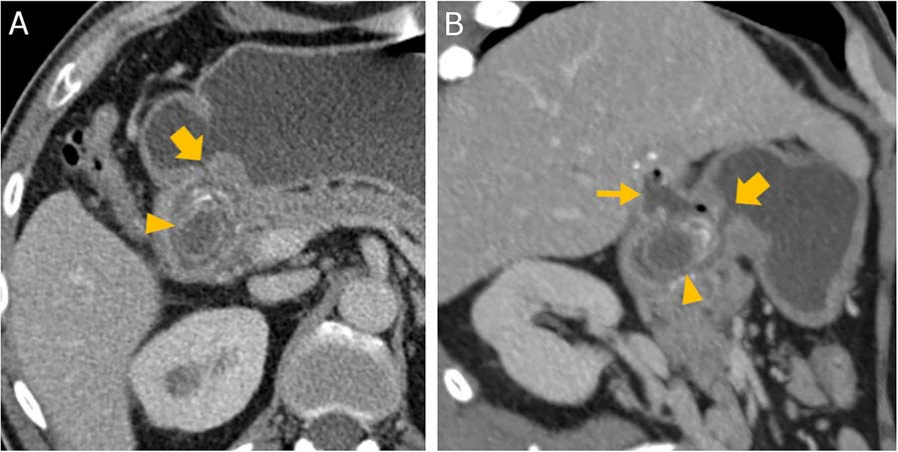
Abdominal CT in axial (A) and oblique parasagittal reconstruction (B); gallstone in the duodenal bulb (arrowhead
